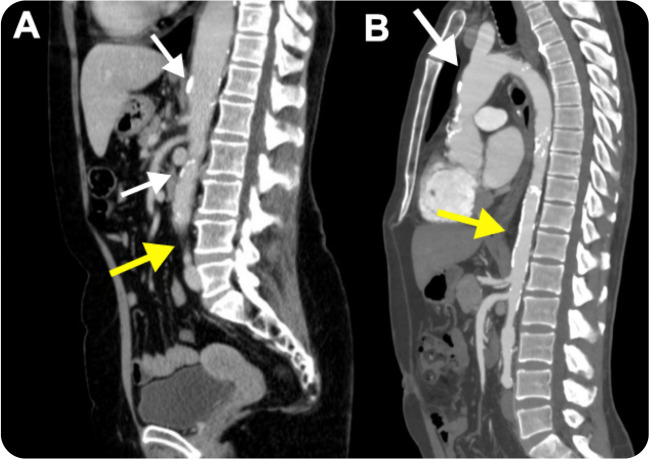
Heterogeneity of aortic involvement in two siblings from the second family. (A) Sagittal view of a CT scan from Case 1 (the 69-year-old sister) showing a different pattern with narrowing of the distal abdominal aorta (yellow arrow) and focal parietal calcifications (white arrows). (B) Sagittal view of a CT angiogram from Case 3 (the 58-year-old brother) showing diffuse parietal thickening and calcification of the aorta (yellow arrow) and ectasia of the ascending aorta (white arrow).
